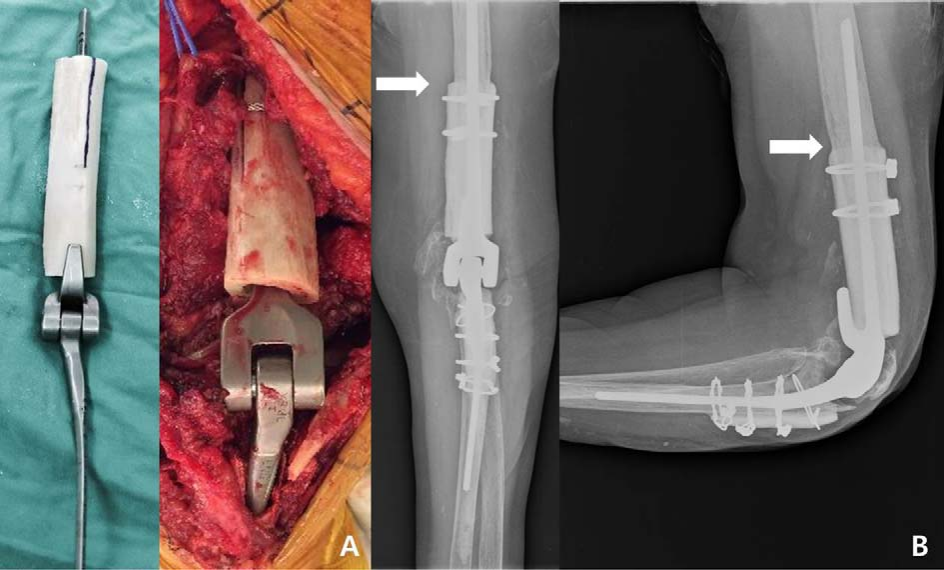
Second-stage revision surgery using APC. (A) Modified type I APC for management of bone defect. (B) Plain radiograph at the time of final follow-up. Bone union was observed between the host and the allo-bone graft.
